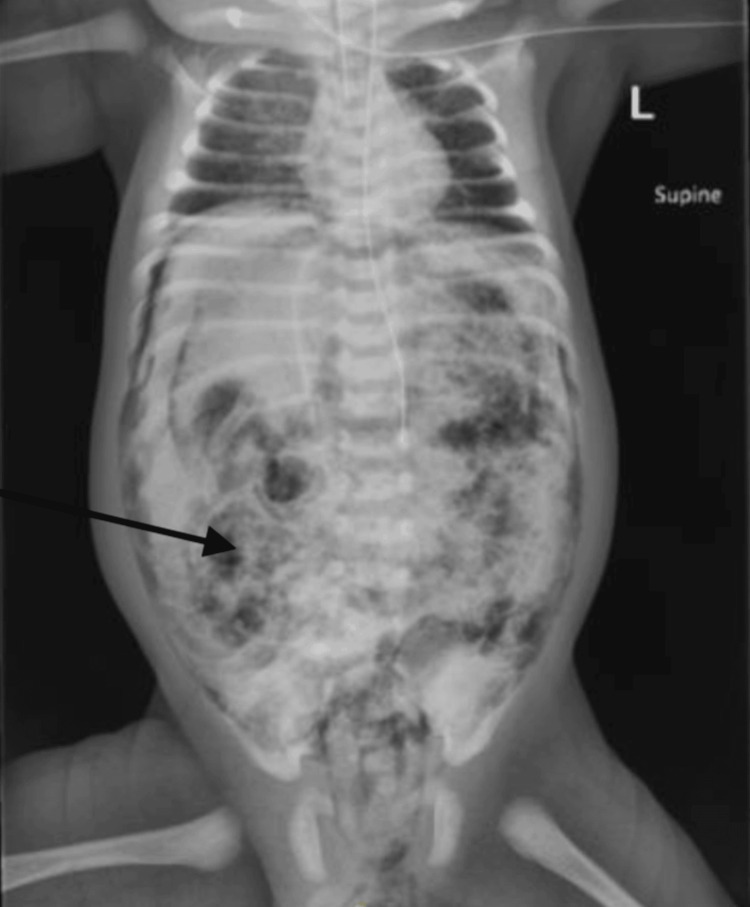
Case 2 - Abdominal X-ray demonstrating extensive pneumatosis intestinalis, i.e., cystic lucency in the walls of the intestines and evidence of free air in the abdomen. The black arrow indicates pneumatosis intestinalis and free air underneath the diaphragm.
